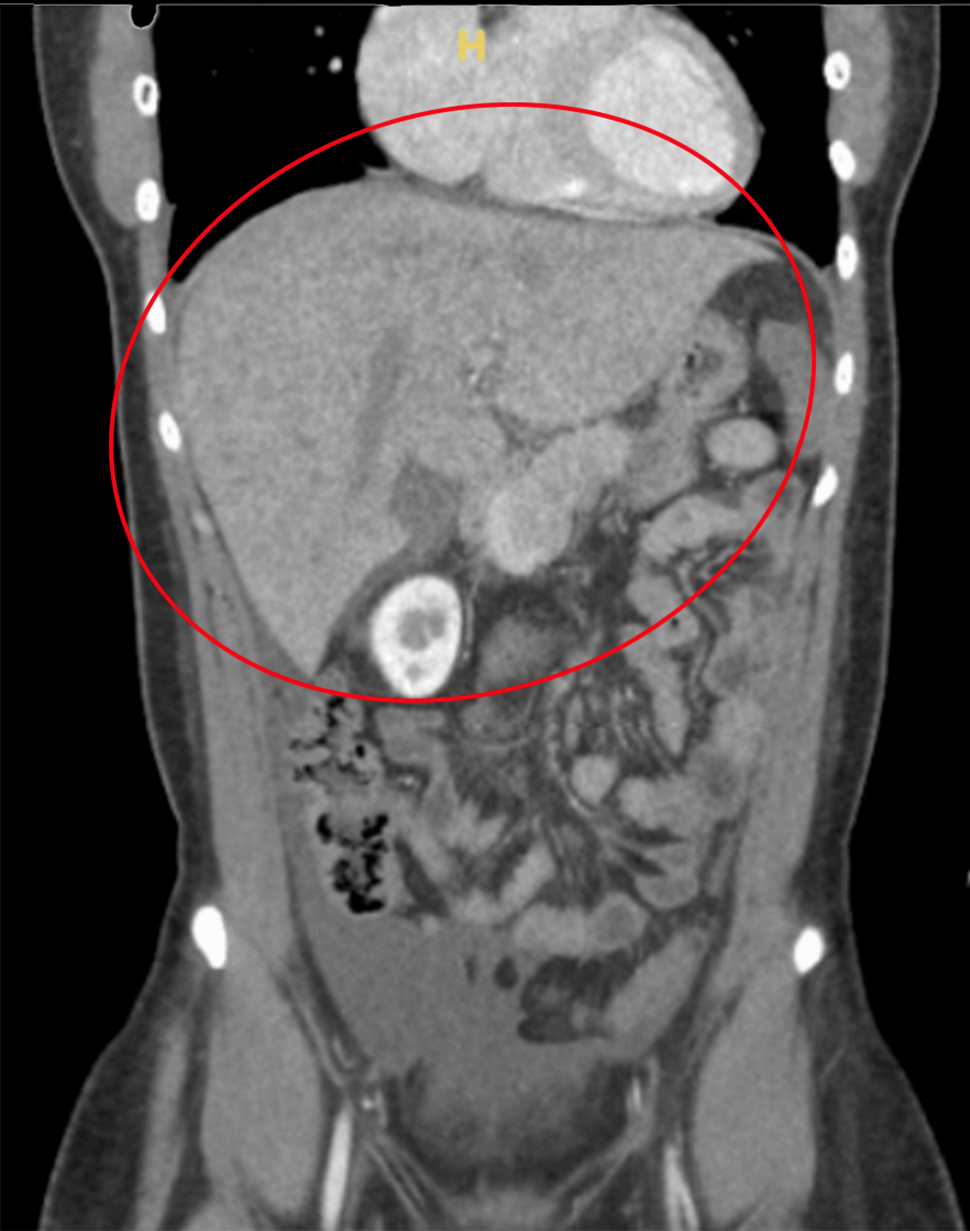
CT scan (coronal section) CT scan of the abdomen and pelvis (CTAP) showing hepatomegaly extending beyond its normal boundaries. There is also a noticeable presence of ascites, with fluid accumulation visible in the peritoneal cavity, surrounding the liver and intestines.
